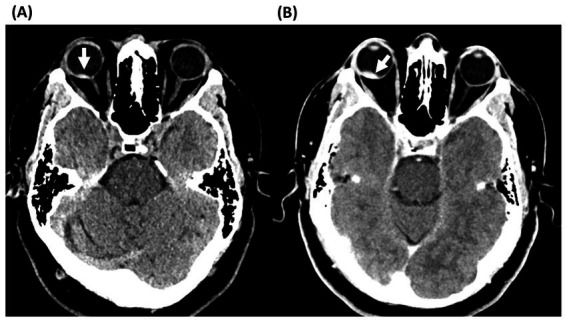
Axial head computed tomography without contrast of case 2. (A) CT head without contrast at initial presentation showing spontaneous intraretinal hemorrhage in the posterior pole of the right eye. (B) CT head after administration of intravenous thrombolysis showing enlargement of the hyperdensity, consistent with worsening of the bleed.
